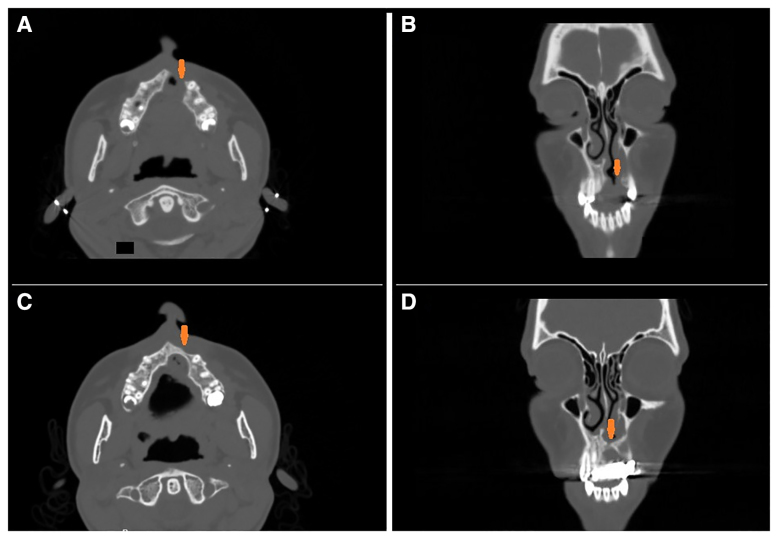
CT images of a patient with cleft lip accompanied by left alveolar cleft before secondary revision and postoperative 12th month. Figure 4 shows the CT scan result of a female patient (20 years old). (A, B) Preoperative tomography image of a patient with a left-sided alveolar cleft, (C, D) CT scan image at 12th month after alveolar cleft repair. CT = computed tomography.
